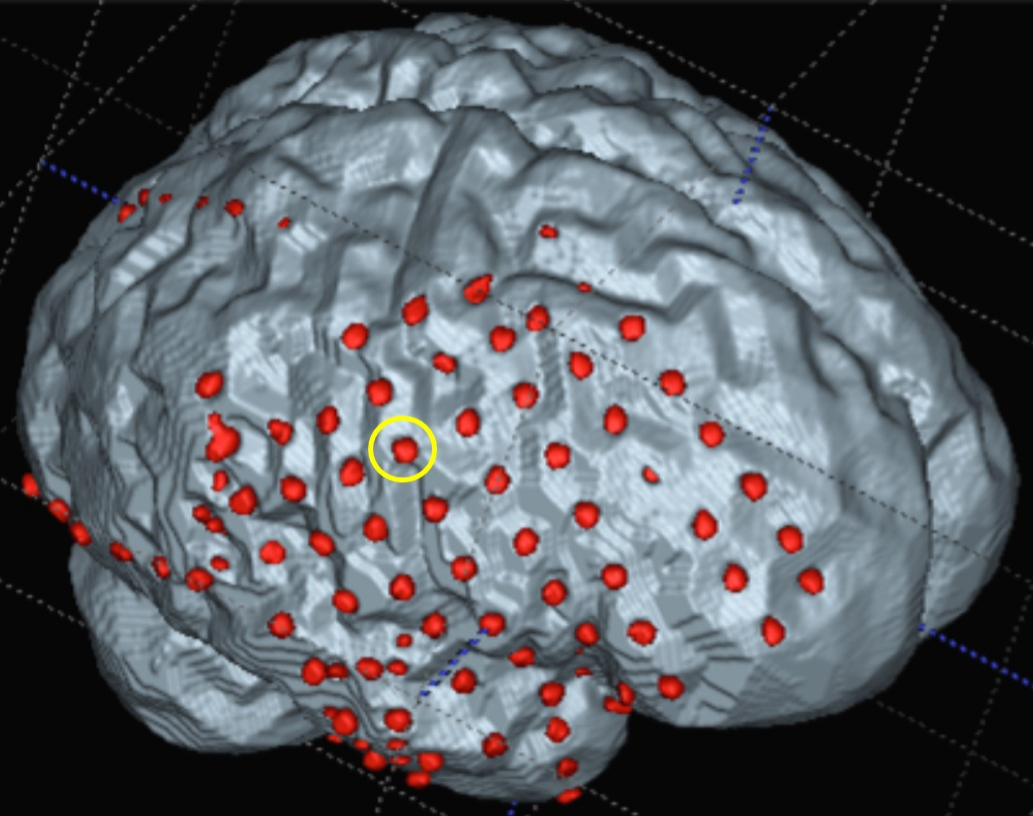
3-D iEEG grid representation Grids superimposed on a three-dimensional magnetic resonance-computed tomography (MR-CT) co-registered surface mask reconstruction of the patient’s cortex. Contact 31 is circled in yellow.
